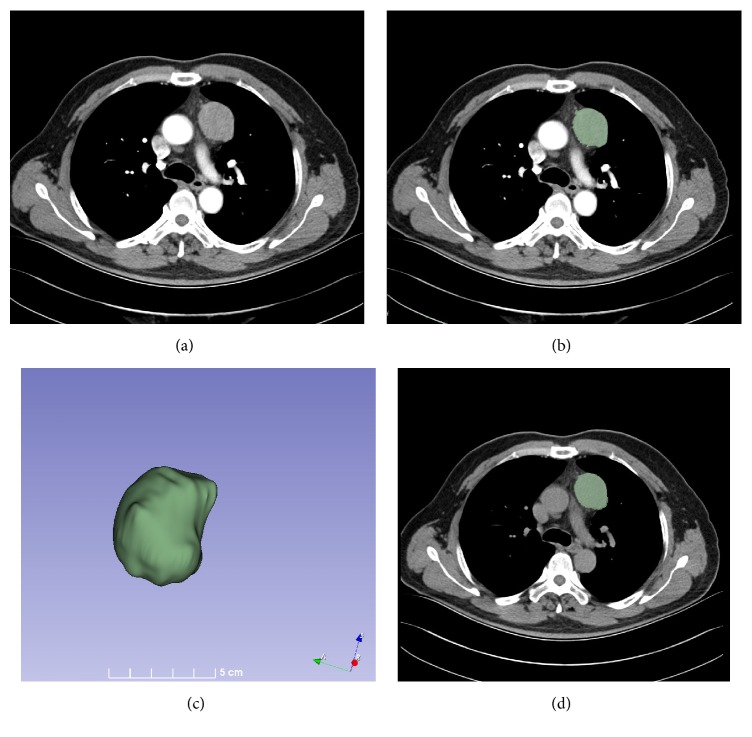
The process of segmentation. (a) Enhanced axial CT scan revealed the presence of a round mass in the left anterior mediastinum. (b) VOIs were drawn in all axial CT images. (c) VOI masks extracted from CT scans. (d) VOI masks were applied to NECT images.
